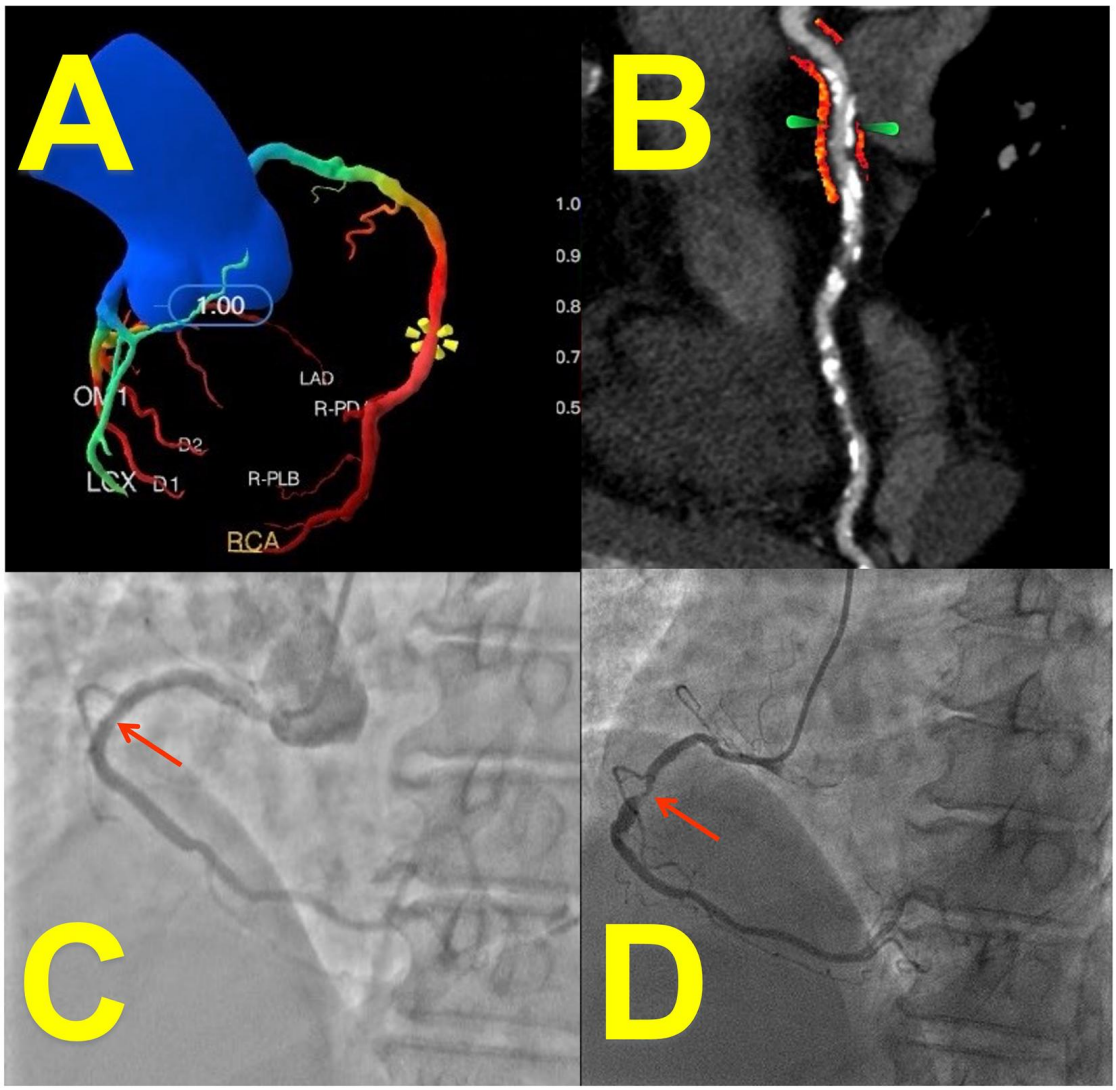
Case presentation: imaging of a 65-year-Old female with coronary artery disease. (A) CT-derived FFR (CT-FFR) measured at stenosis of right coronary artery (RCA): 0.58 (deep-learning reconstruction). (B) Peri-coronary fat attenuation index (FAI, arrow; red area: fat within −190 to −30 HU range) at stent site: −67 HU (curved-planar CCTA reconstruction). (C) Patent stent lumen confirmed on angiography 1 month post-PCI. (D) Invasive coronary angiography (ICA) at 16-month follow-up revealed significant in-stent restenosis (ISR, arrow) corresponding to the patient's recurrent exertional chest tightness (relieved within 5 min of rest).
